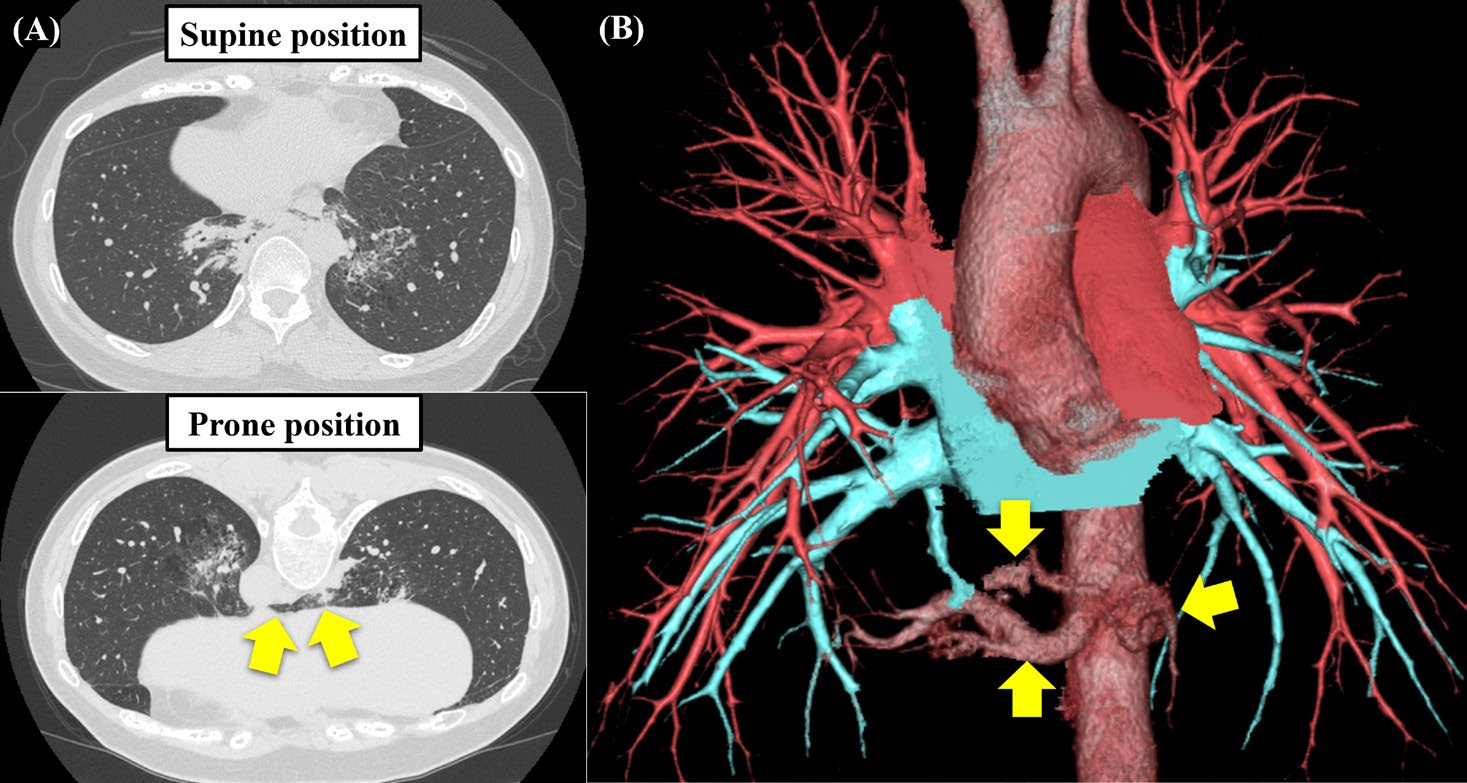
Preoperative chest computed tomography findings. (A) Imaging in the prone position visualizing the horseshoe lung, in which the left and right lungs were fused at the isthmus (arrows). (B) Three aberrant arteries originate from the descending aorta (arrows), whereas the pulmonary veins drain into the normal inferior pulmonary veins on both sides. The computed tomography images were reconstructed in three‐dimensional form with the Ziostation software (Ziosoft Inc., Tokyo, Japan).
